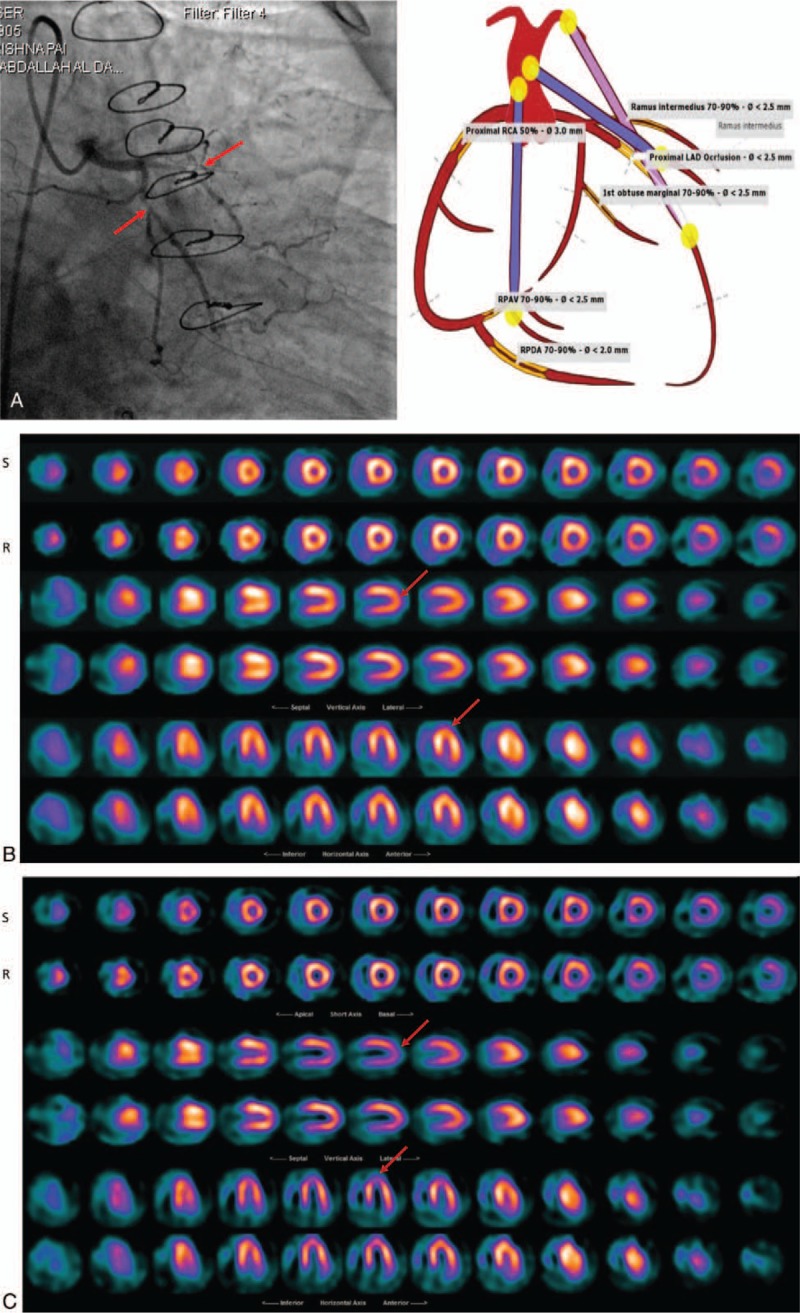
A 53 years old male patient with the history of CAD was refereed to NM for evaluation of myocardial perfusion post CABG. (A): Angiography result reported triple vessels disease, (B): Butterworth reconstructed SPECT results reported mild degree of ischemia in apex, (C): Wiener reconstructed SPECT results suggested severe diffuse uptake in anterior and anterolateral walls with moderate diffuse uptake in apex that was more consistent with angiography. CABG = coronary artery bypass grafting, CAD = coronary artery disease, SPECT = single photon emission computerized tomography.
